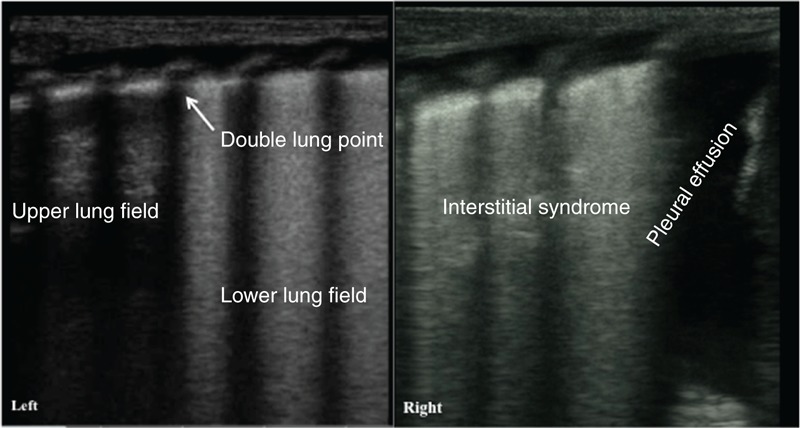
Ultrasonic manifestation of TTN: pleural effusion. The infant above had a GA of 39 wk and was delivered by cesarean section; the infant had a birth weight of 3980 g. The infant was admitted to the hospital after suffering from dyspnea for 5 h. Lung ultrasonography showed the disappearance of the pleural lines in both the lungs, A-lines, and interstitial syndrome in the left lung field and pleural effusion in the right lung. This case also demonstrated that the degree of the injury in each of the lungs in a child with TTN could be different. GA = gestational age, TTN = transient tachypnea of the newborn.
